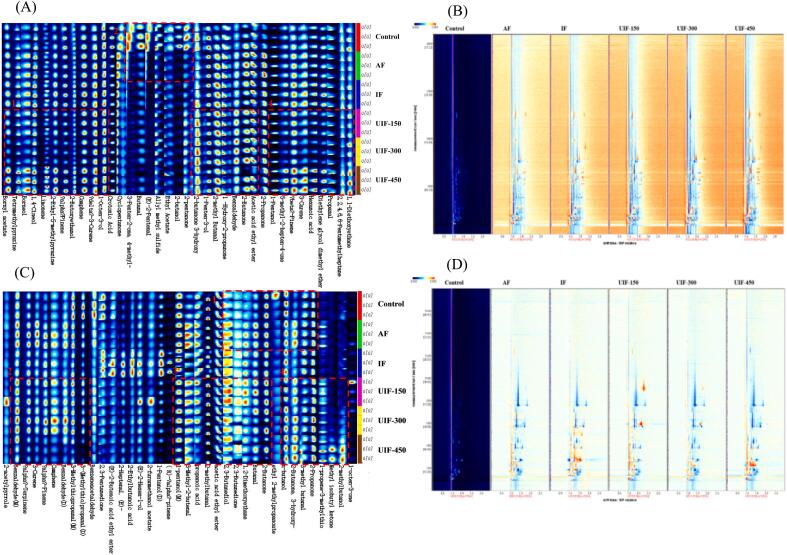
Fingerprint of volatile compounds and two-dimensional map of volatile compound of beef (A and B) and potatoes (C and D) in braised beef with potato dishes under different freezing treatments. AF, air freezing; IF, immersion freezing; UIF, ultrasound-assisted immersion freezing at different ultrasound powers (150, 300 and 450 W). “M” and “D” denote monomer and dimer, respectively.
